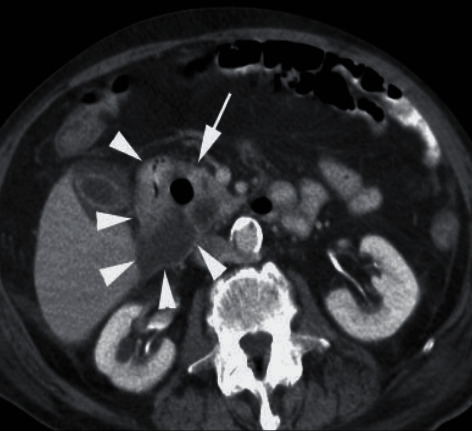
Duodenal diverticulitis with retroperitoneal perforation: (a) coronal CT scans at admission, (b) after 8 days of conservative treatment, and (c) after six weeks. The retroperitoneal air slowly resolves while the inflamed duodenal diverticulum (arrow) regains its normal aspect.
